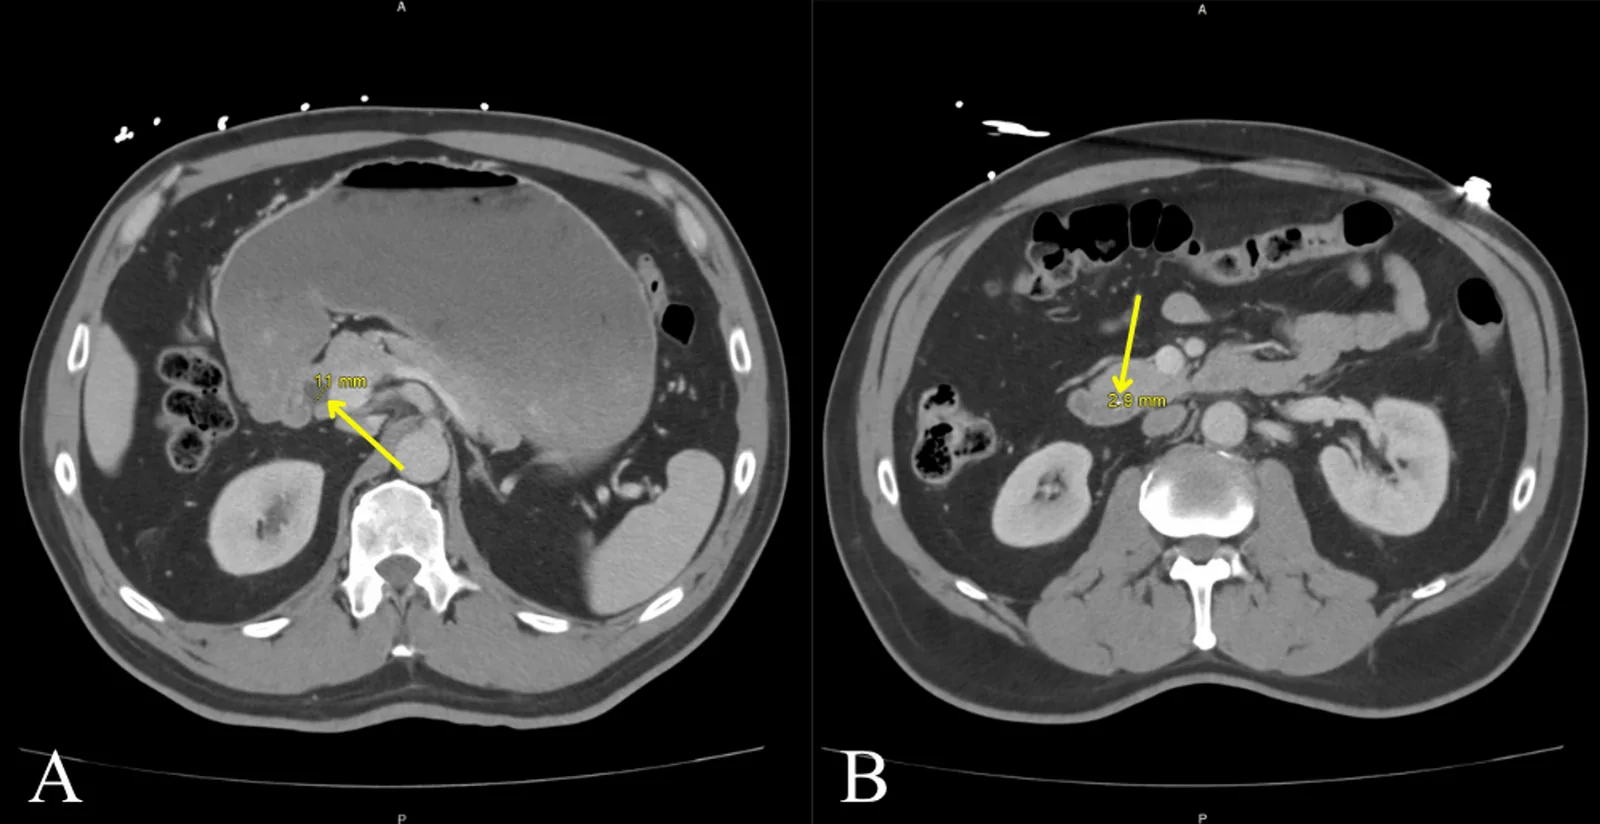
Axial contrast‑enhanced CT of the abdomen demonstrating choledocholithiasis and mild intrahepatic bile duct dilatation (Panel A) The arrow marks a calcified stone at the distal common bile duct near the ampulla, measuring approximately 11 mm. (Panel B) The arrow indicates a more proximal common bile duct stone measuring approximately 2.9 mm, with associated mild prominence of the extrahepatic bile duct, consistent with the reported choledocholithiasis.
